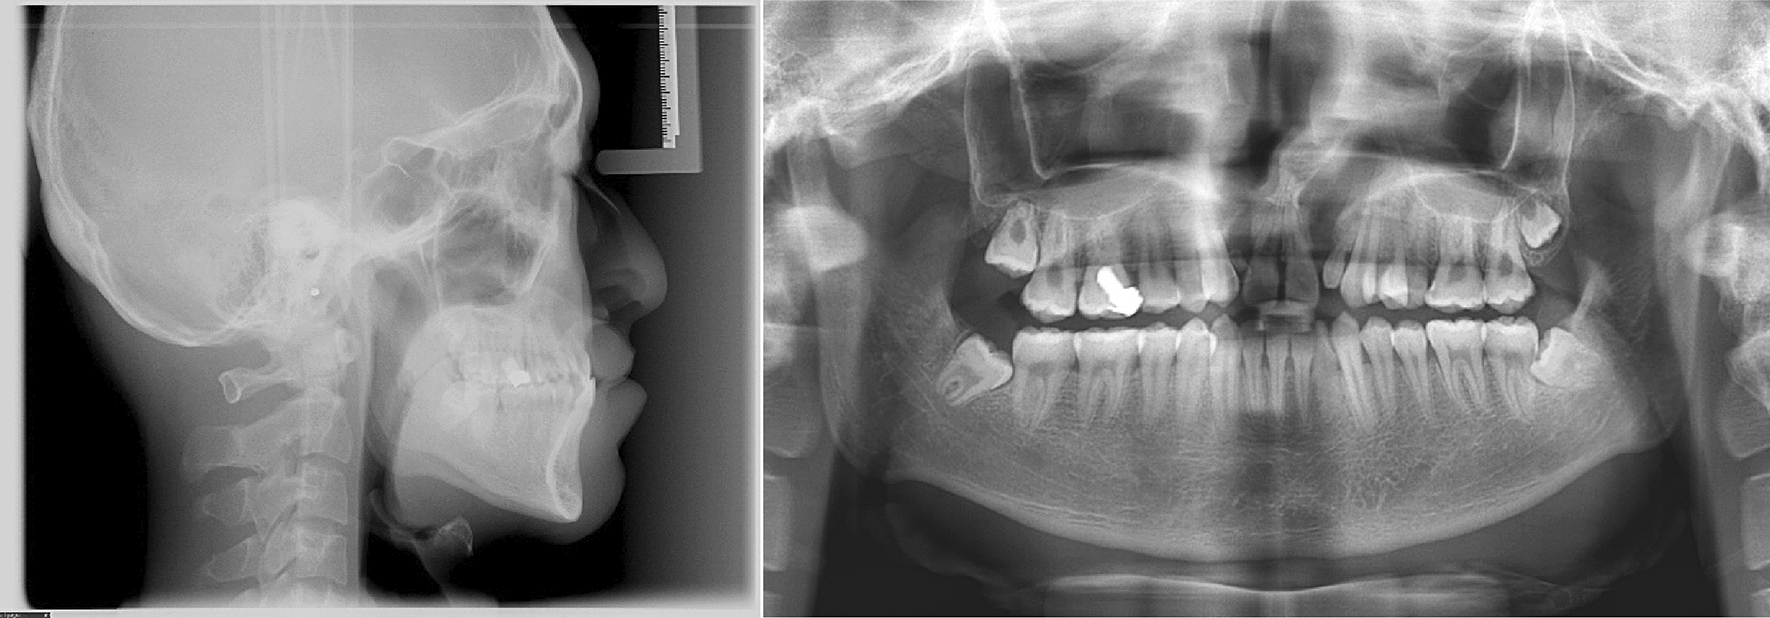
Pretreatment lateral radiograph and panoramic radiograph
